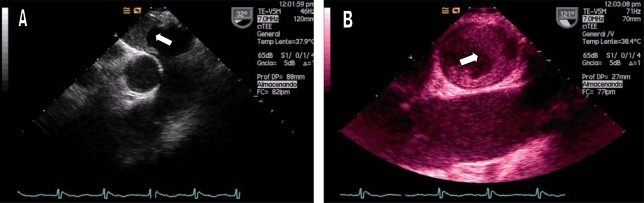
Patient in shock state, with high suspicion of septic shock due to cholecystitis (fever, right hypochondrium pain). In the transesophageal study we found a giant thrombi in the right pulmonary artery (RPA). A) TEE for 30°. B) TEE for 120°.
